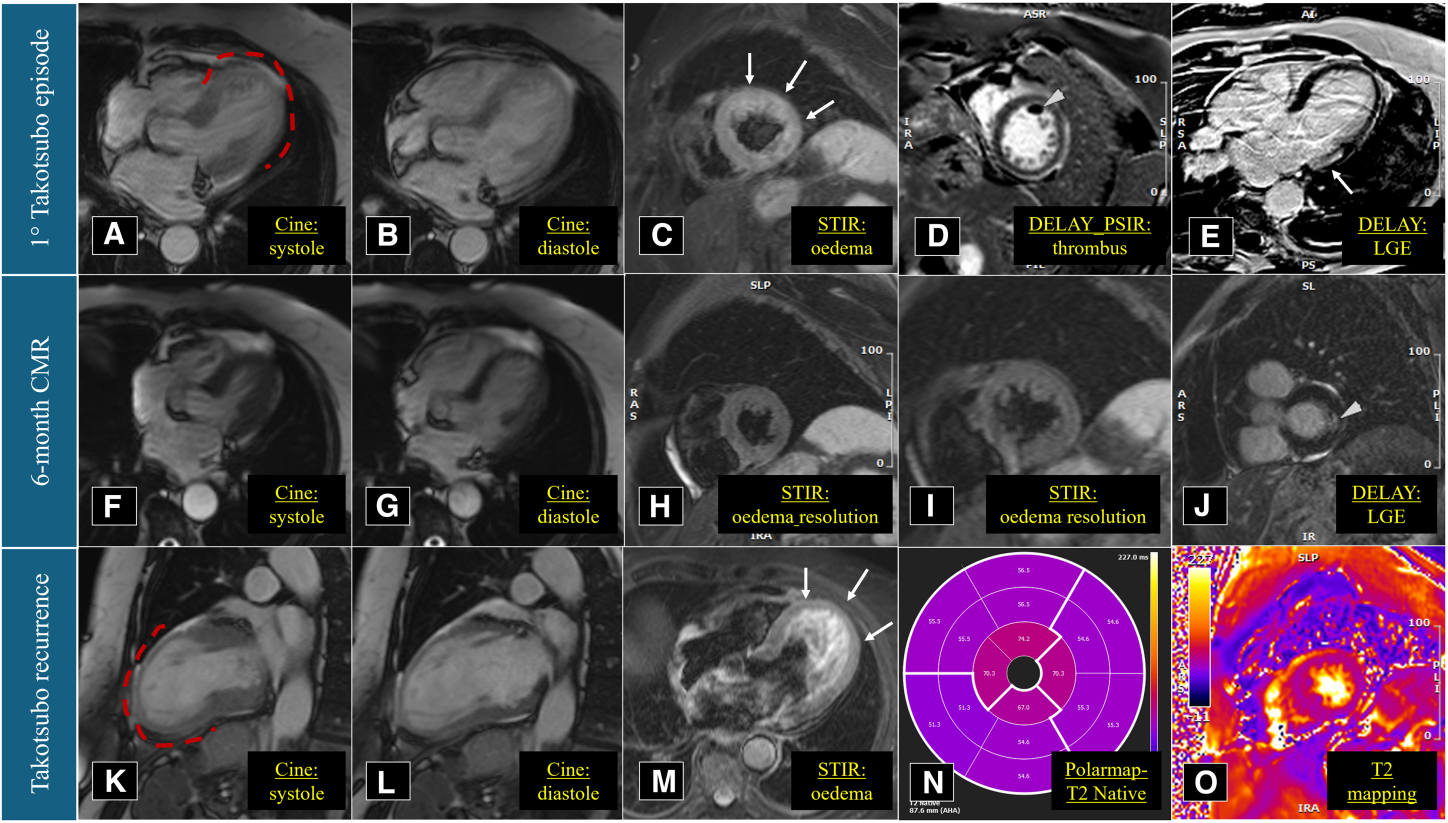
Cardiac magnetic resonance imaging performed in March 2021 (A–E), September 2021 (F–J), and March 2023 (K–O). Red dotted lines underline the apical akinesia during left ventricular systole.
